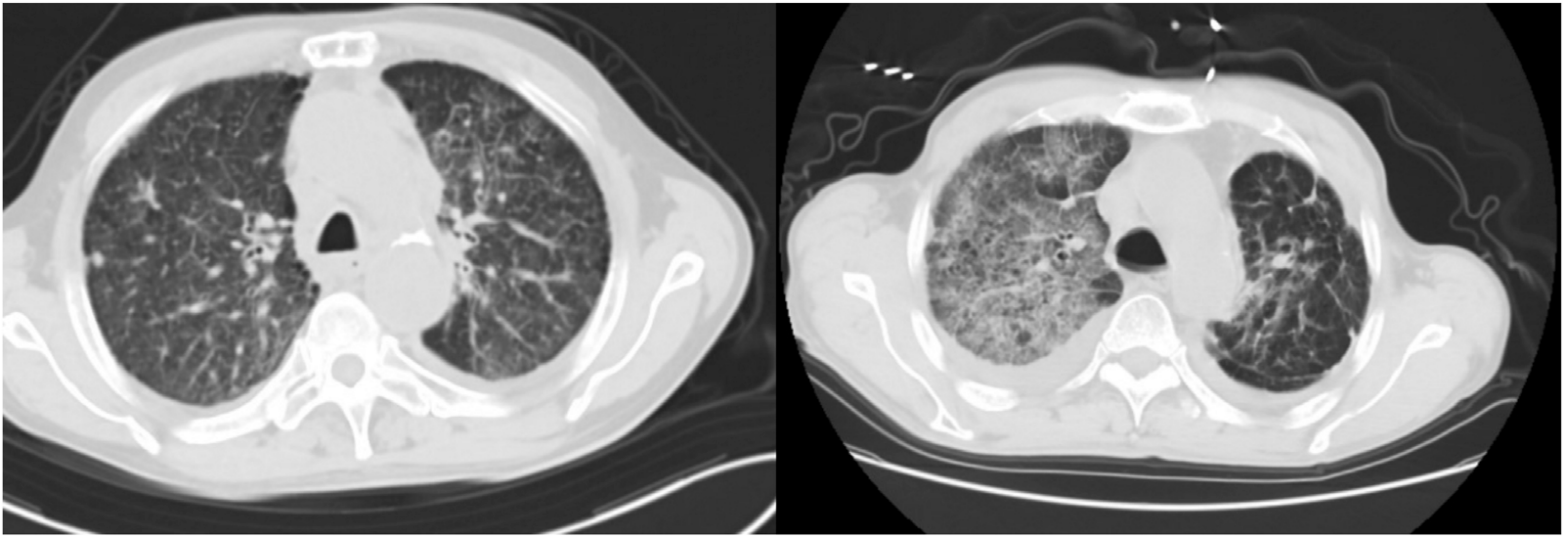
Chest HRCT of case 1 and case 2.
